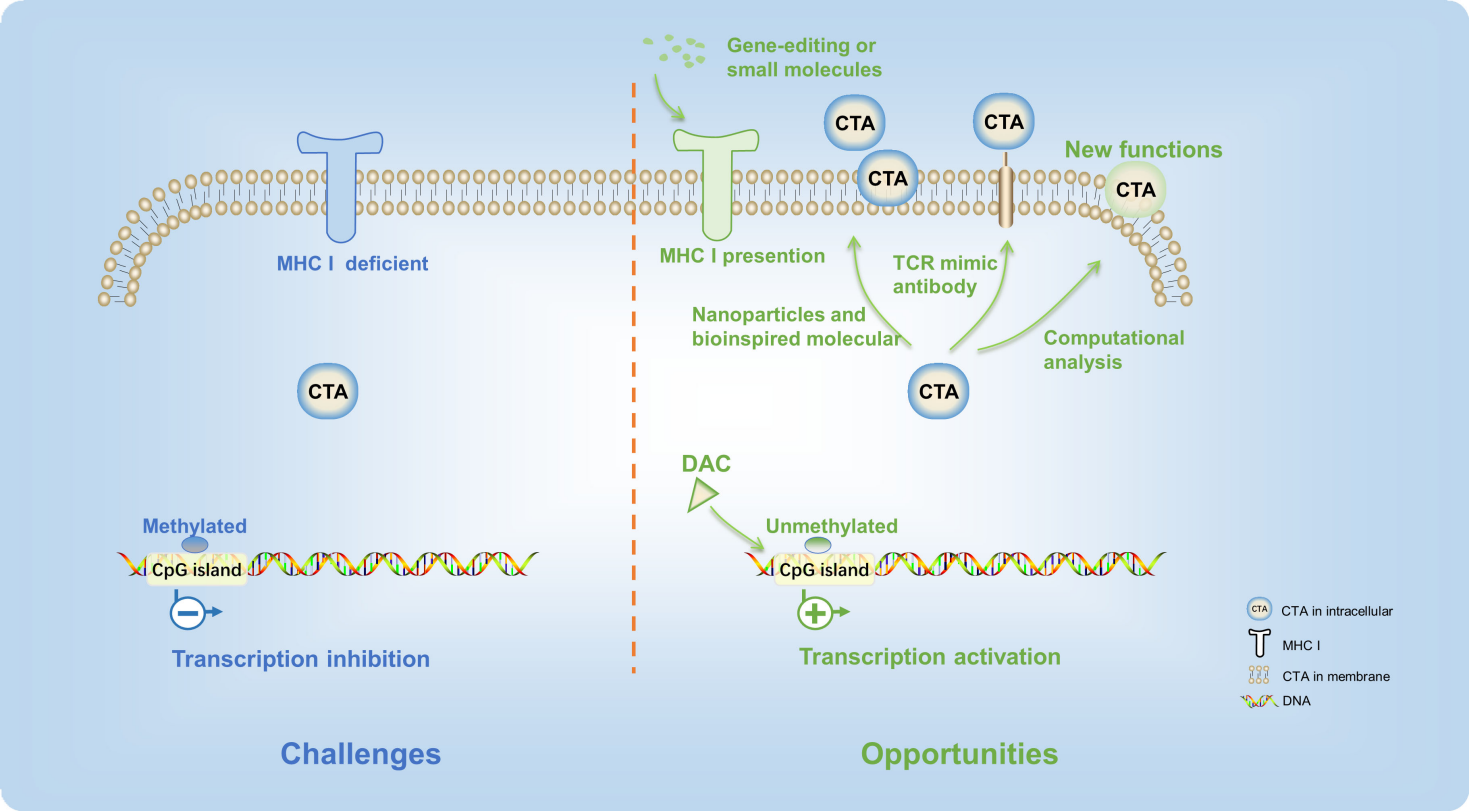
There are many detours and challenges in the CTA-based digestive tract tumor immunotherapy. New strategies and cutting-edge technology provided opportunities to overcome these difficulties.
